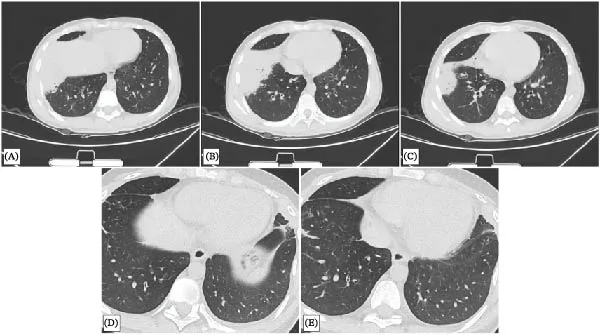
Chest CT images at presentation. Subfigures (A–C) show consolidation involving the right middle and lower lobes, including the posterior basal segment, consistent with pneumonia. No cavitation or bronchiectasis was observed. Subfigures (D, E) show follow‐up chest CT images obtained 1 month after discharge, demonstrating near‐complete resolution with no significant residual lesion in the right lower lung.
